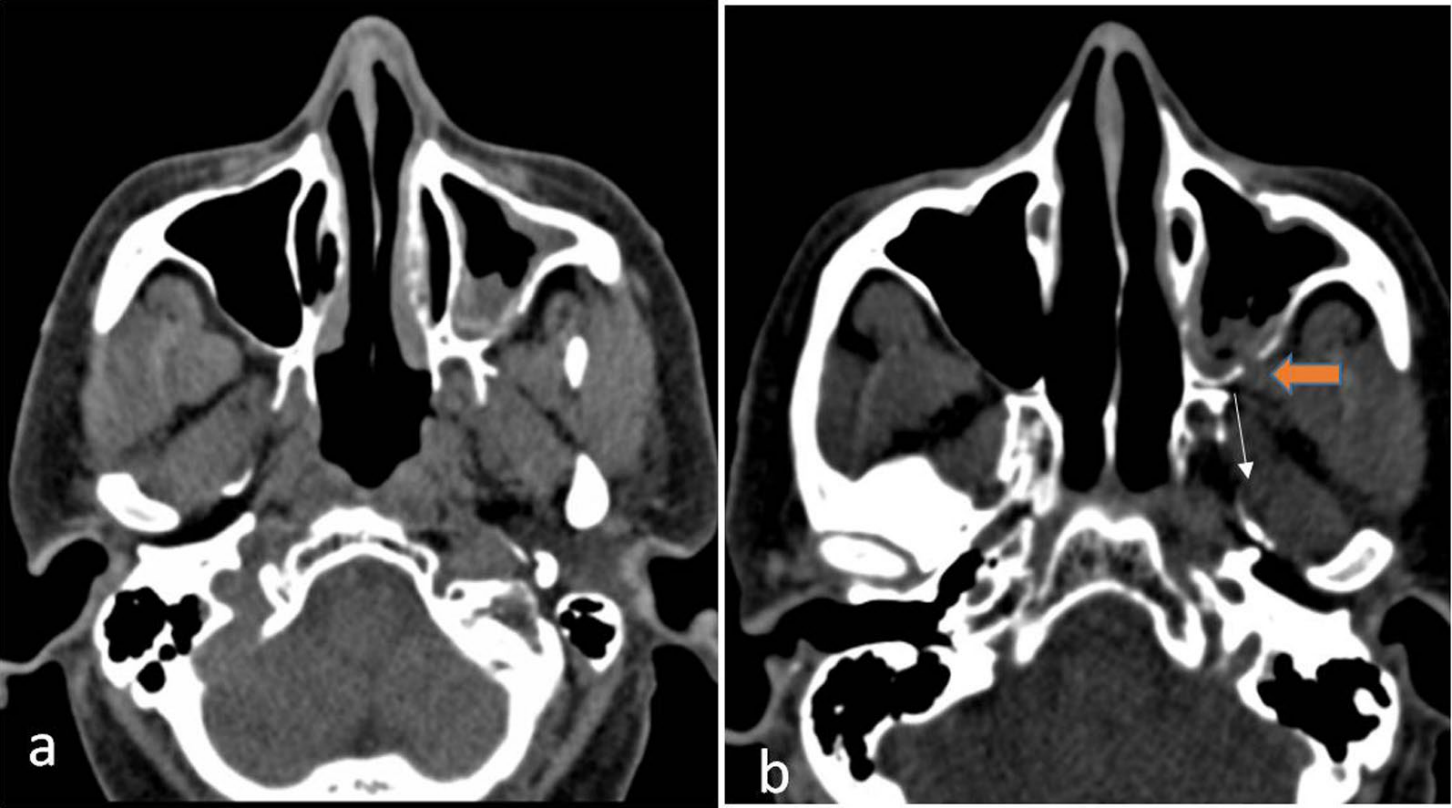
In a 35-year-old patient post COVID-infection of moderate severity, presented with sinusitis. Axial CT images (a, b) showing left maxillary sinusitis with the erosion of the posterior wall of the left maxillary sinus (orange arrow) and obliterating the retroantral fat, consistent with invasive fungal sinusitis
